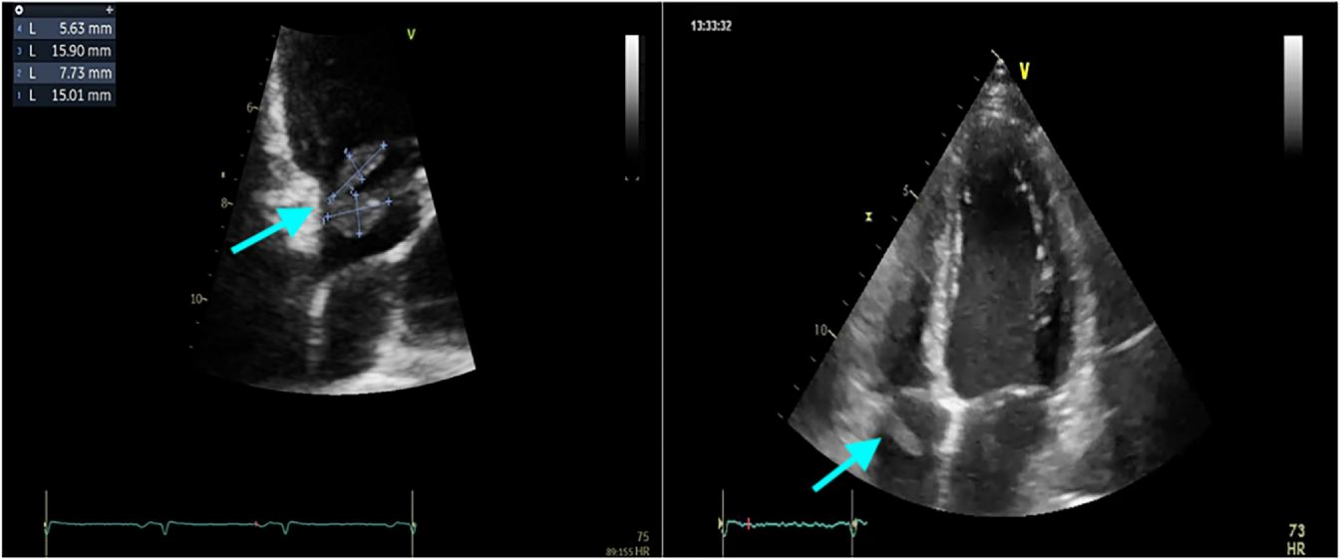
Images 1 and 2: transthoracic echocardiography at admission showing a mobile pediculated bilobed masse of diameter 9 × 14 mm and 6 × 14 mm attached to the lateral side of the tricuspid annulus within the right atrium in favour of vegetation and mild tricuspid regurgitation (see arrows).
